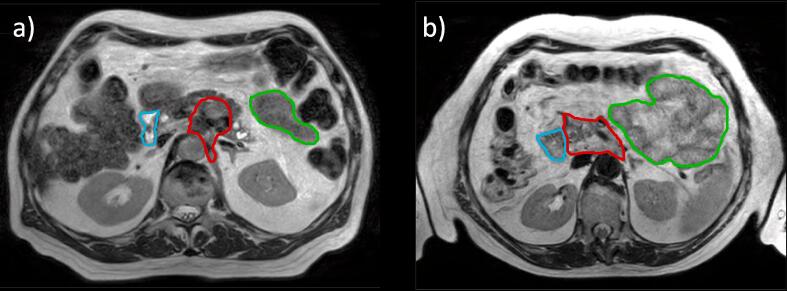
3D *T*_2_w MRI data of case 1 (a) and case 2 (b), on which the planning contours are projected (red = GTV, cyan = duodenum, green = small bowel). Note that not all contours are shown and that treatment planning itself was performed on CT imaging. (For interpretation of the references to colour in this figure legend, the reader is referred to the web version of this article.)
